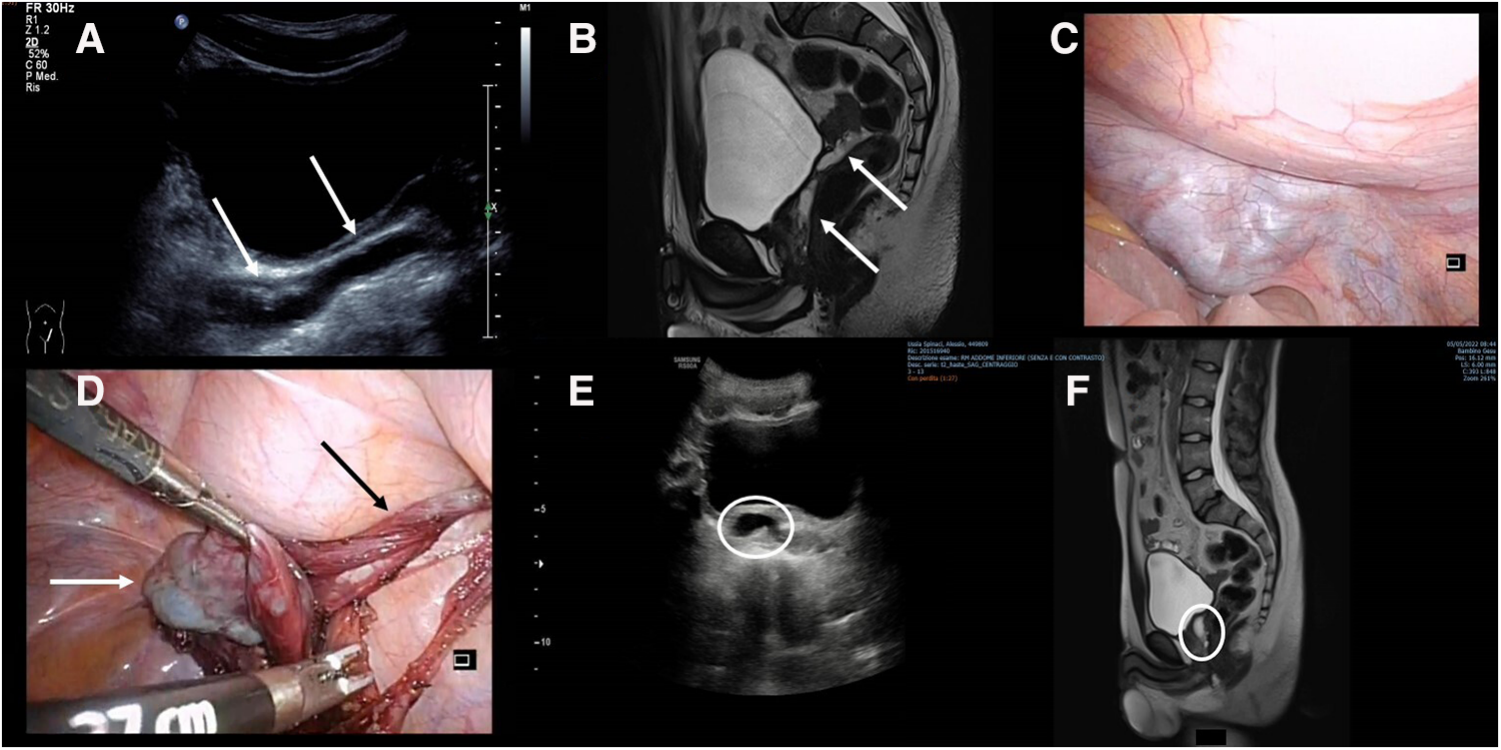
(A) US showing the presence of a 10 mm × 50 mm unilocular dilatation of the seminal vesicle (white arrows); (B) MRI confirming the presence of the retrovesical cyst (white arrows); (C) laparoscopic view of the cystic lesion; (D) intraoperative view of the SVC (white arrow) and vas deferens (black arrow); (E) postoperative US showing the residual SVC; (F) MRI showing the sagittal view of the residual SVC.
